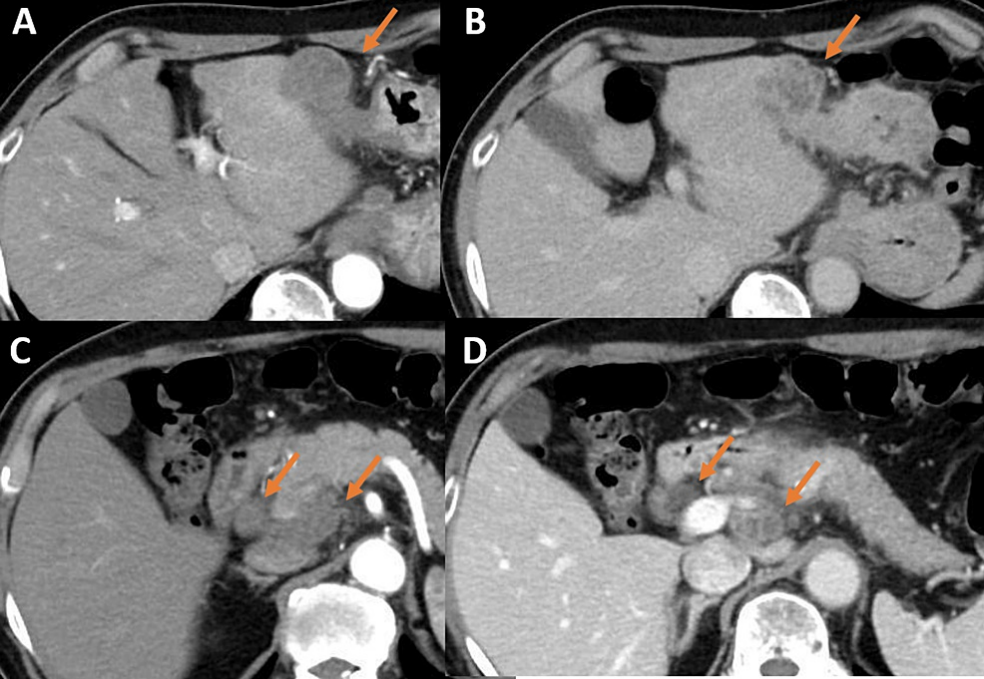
Abdominal contrast-enhanced CT An internal low-concentration mass between the liver and stomach in the arterial phase (A) and the delayed phase (B). Enlarged intra-abdominal lymph nodes around the portal vein in the arterial phase (C) and the delayed phase (D).
